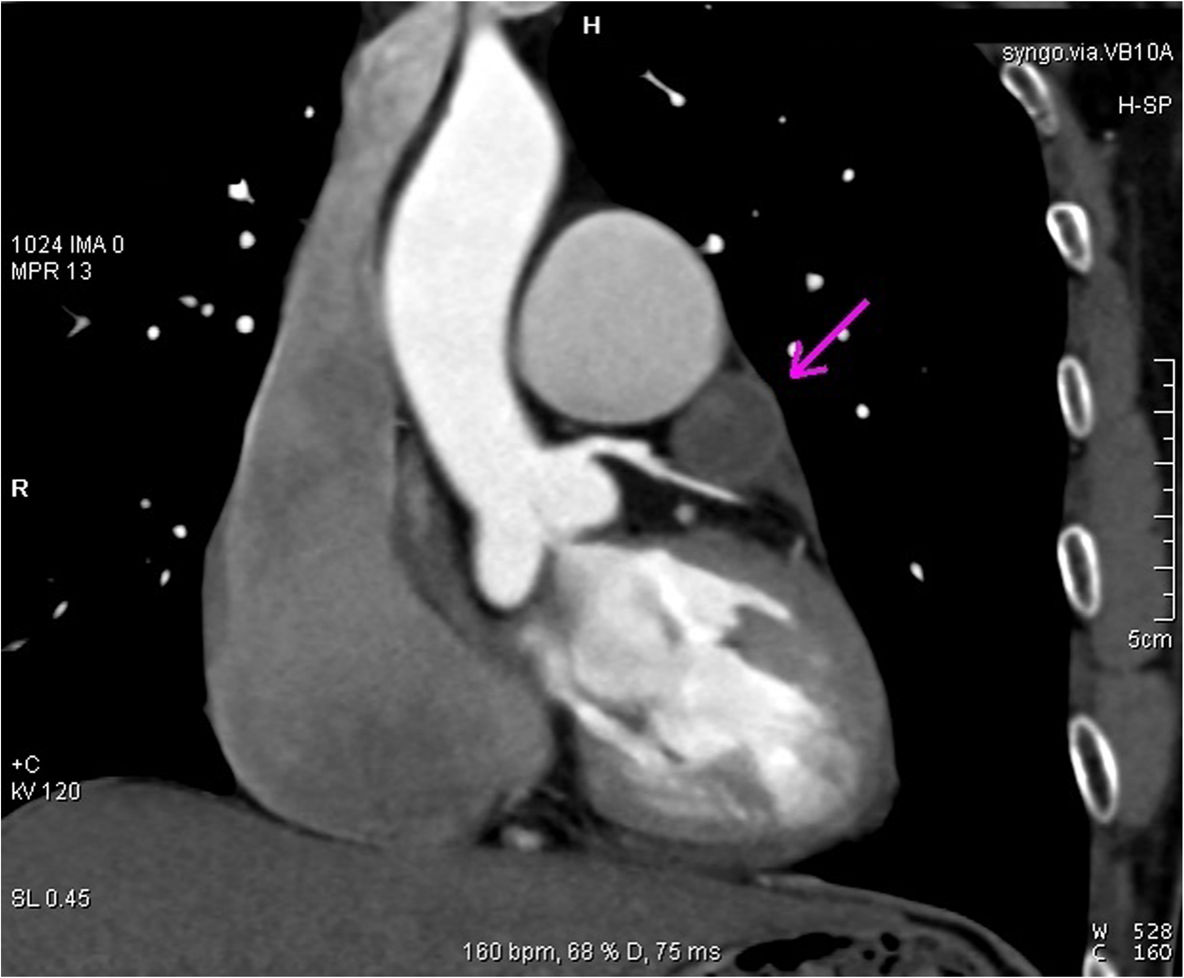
Cardiac 2D CTA reconstruction. The purple arrow points to the mass
